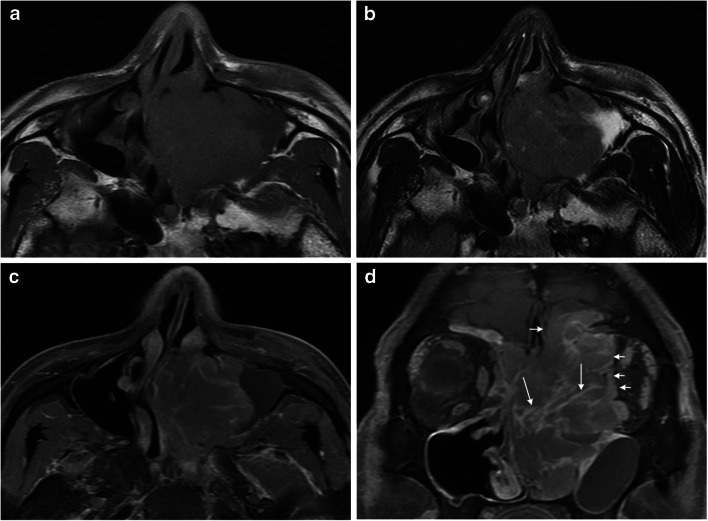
MRI features in a 46-year-old man with diffuse large B cell lymphoma in the sinonasal region. a Axial T1-weighted MR image shows a homogeneously isointense mass in left nasal cavity and maxillary sinus. b Axial T2-weighted MR image shows the tumor with homogeneous isointensity. c, d Axial (c a) and coronal (d) contrast-enhanced T1-weighted MR images show heterogeneous enhancement of the tumor with the septal enhancement pattern (small long arrows) and the involvement of the skull and left orbit (small short arrows)
